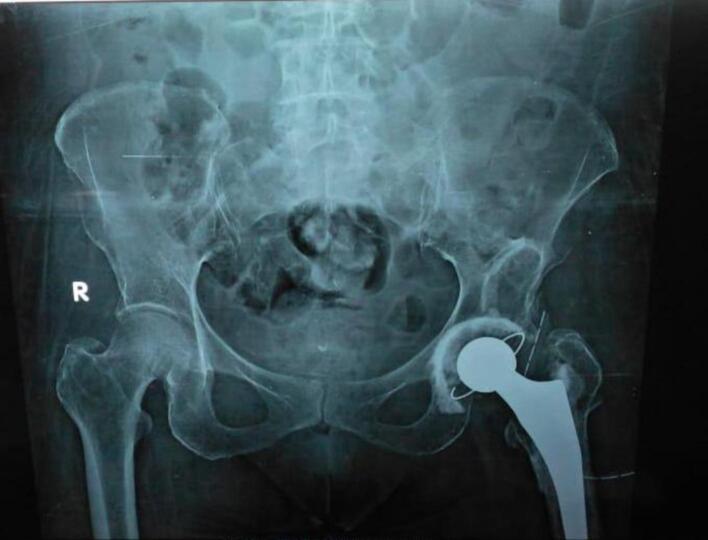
The plain radiograph shows left total hip replacement after surgery.
